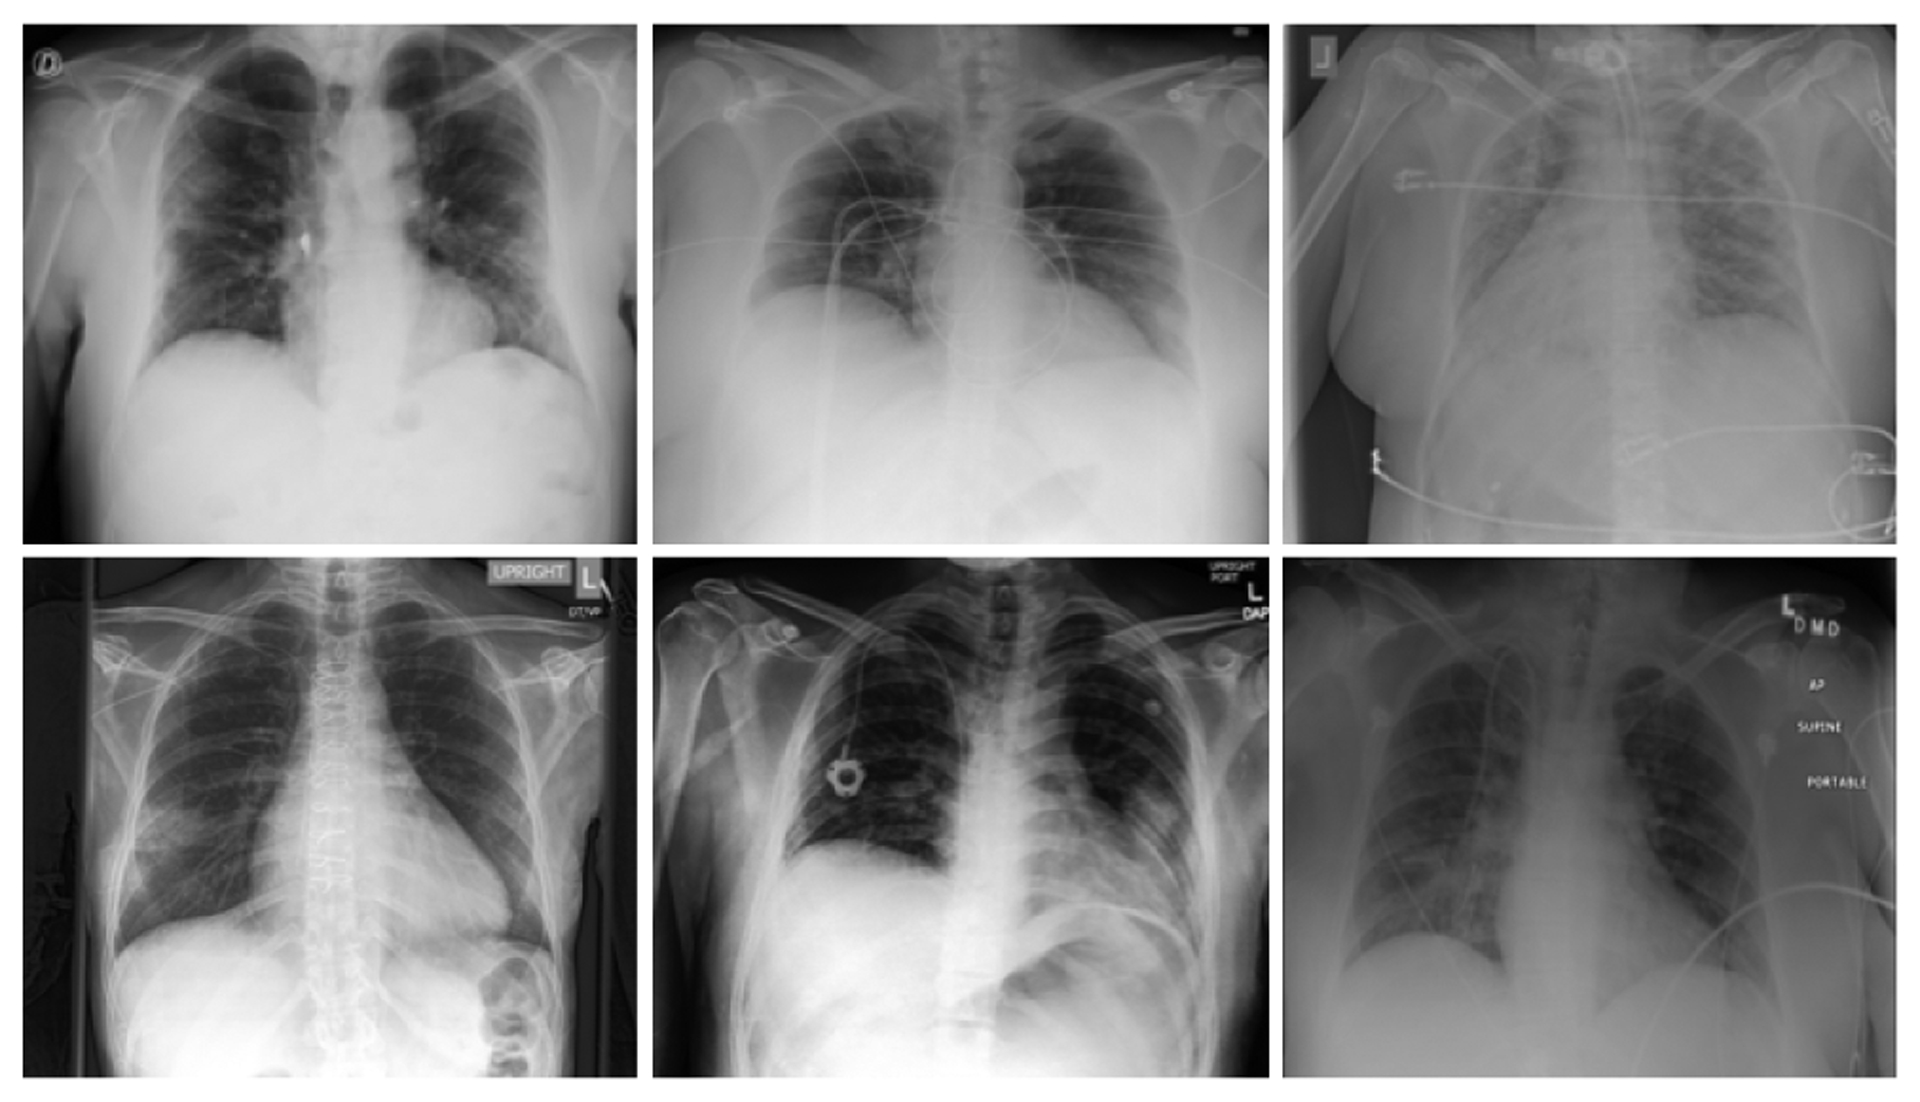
Samples of the input X-rays. TOP: COVID-19 cases. BOTTOM: Pneumonia cases.
